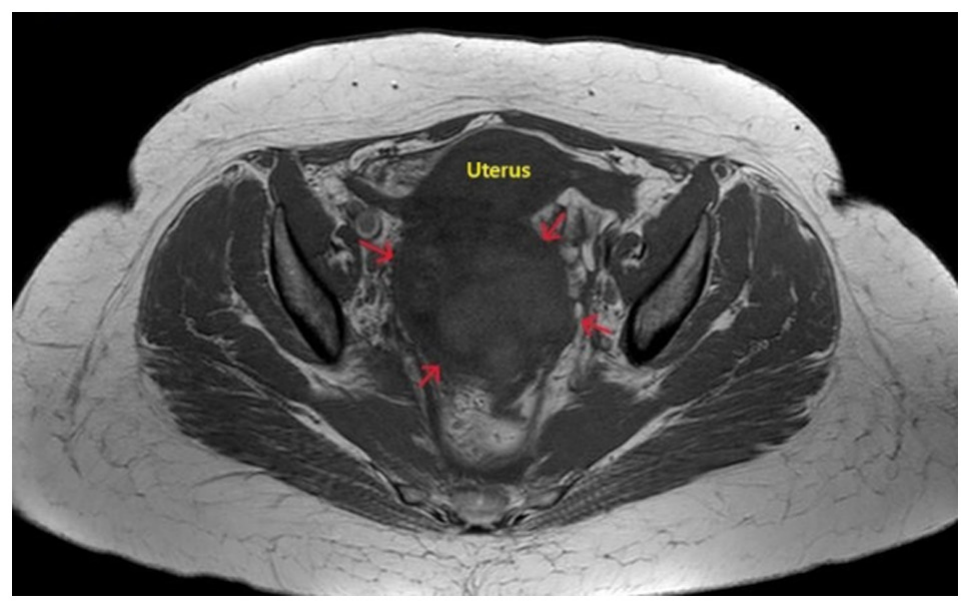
Magnetic resonance imaging of a twisted large ovarian fibroma: A large heterogeneous lesion (red arrows) is clearly visible in the region of the right adnexa, with cystic and necrotic areas present within.
